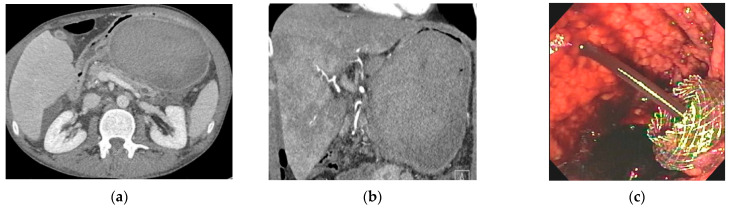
CT and endoscopic image of a hemorrhagic pseudocyst. (a) Sagittal CT imaging of pseudocyst with heterogenous material consistent with hemorrhage; (b) coronal CT imaging of hemorrhagic pseudocyst prior to LAMS placement; and (c) endoscopic imaging post-LAMS placement with blood tinged fluid draining in the stomach.
